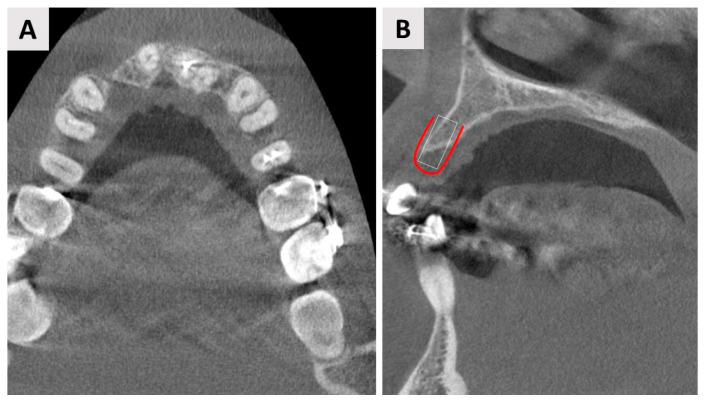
Axial (A) and coronal (B) sections from the CBCT before surgery showing significant bone loss in the horizontal and vertical dimensions. (B) The coronal section shows the planned direction for implantation (white rectangle) and the site designated for regeneration (red line).
